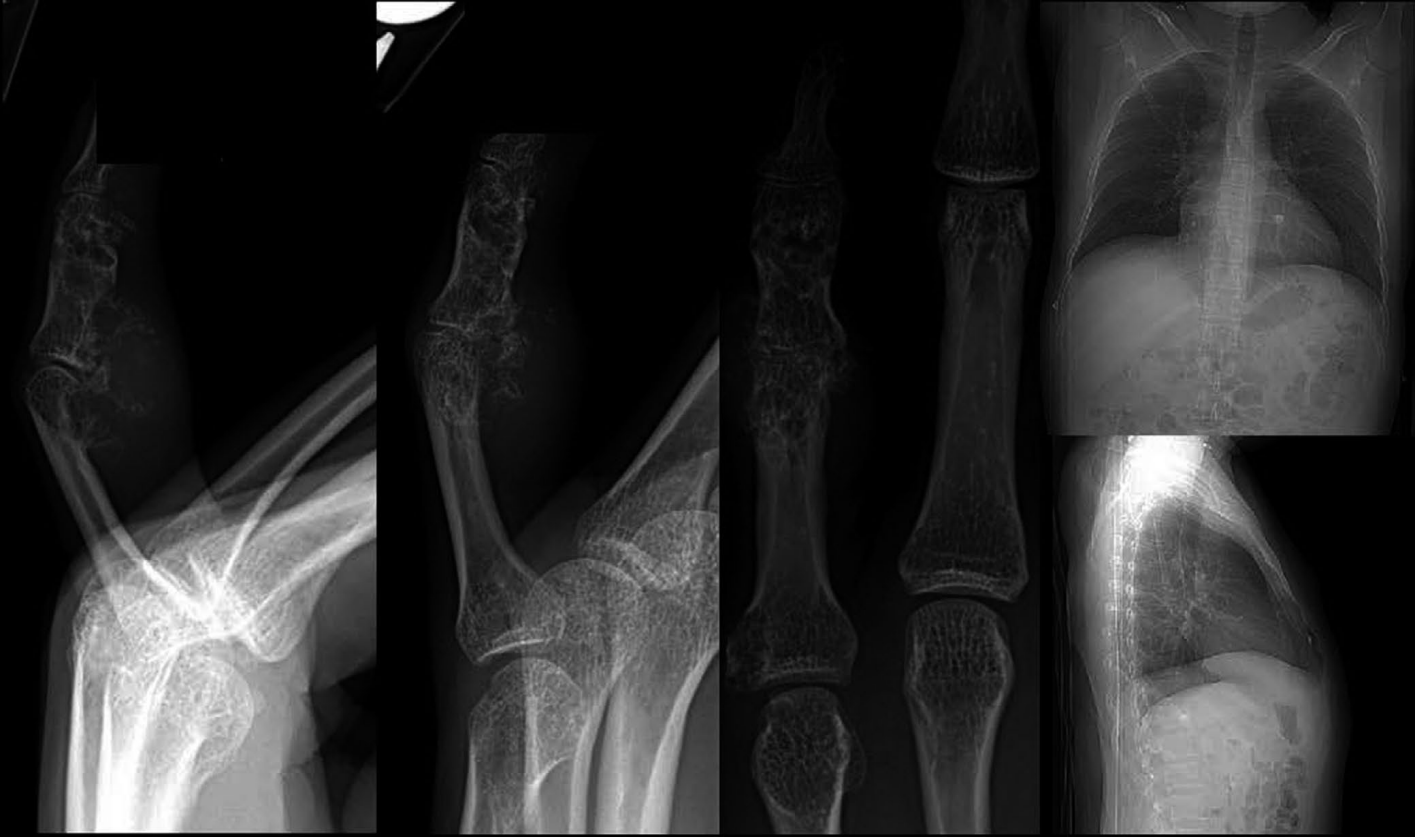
Preoperative radiographs showing abnormality in the small finger
